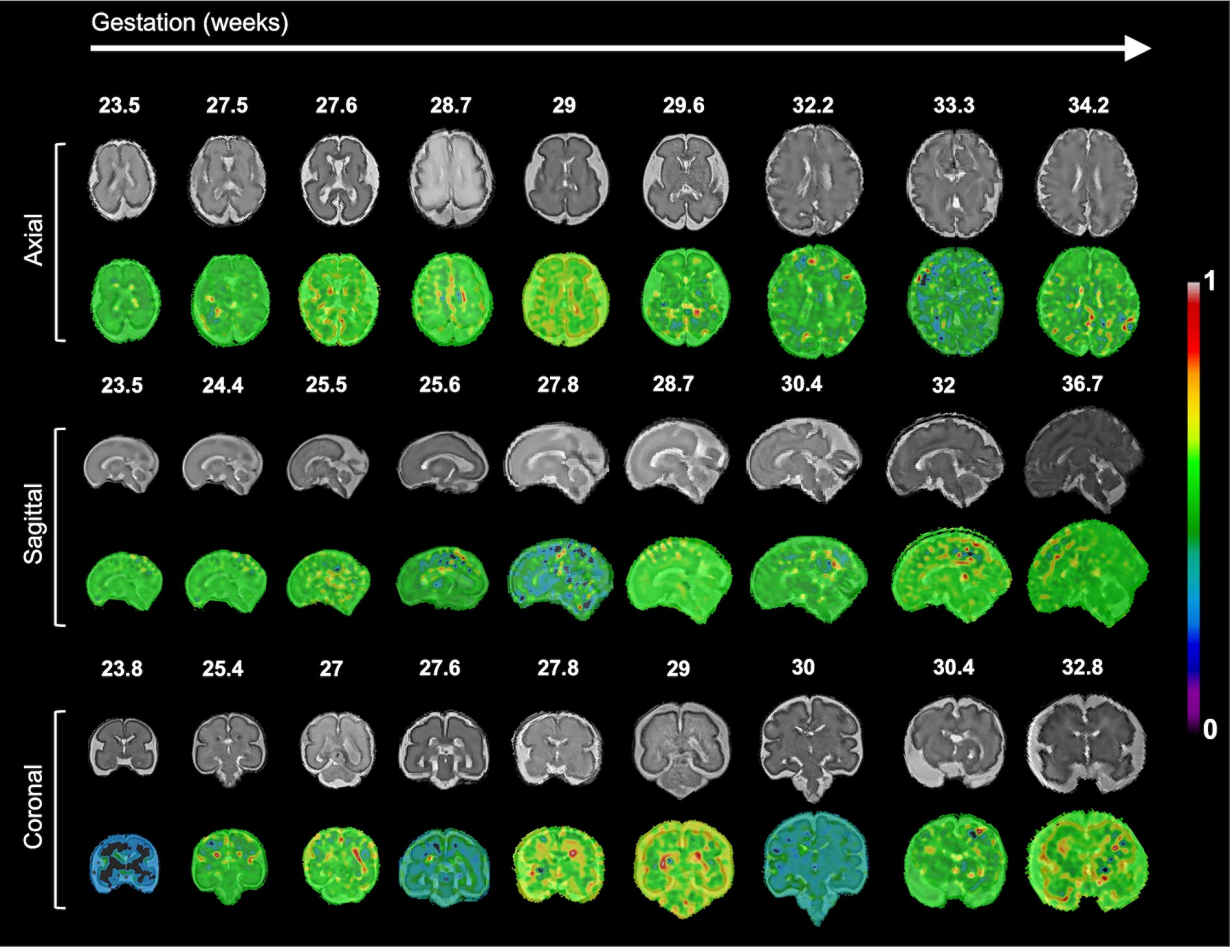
Examples of the saliency map for deep CNN-based brain age prediction model on input 2D MRI slices. The high saliency values indicate that those regions most contribute to the brain age prediction.
